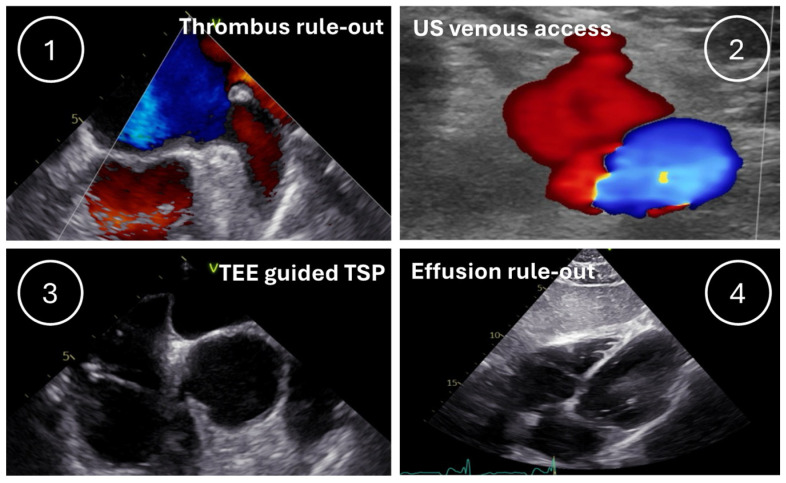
The UltraSound-guided „4“ ABLation strategy (US4ABL): 4 consecutive steps for left-sided ablations. Step 1: left atrial thrombi rule-out using TEE; Step 2: US-guided femoral access; Step 3: US-guided transseptal puncture; Step 4: pericardial effusion rule-out using TTE. US—ultrasound; TEE—transesophageal echocardiography; TTE—transthoracic echocardiography; TSP—transseptal puncture.
